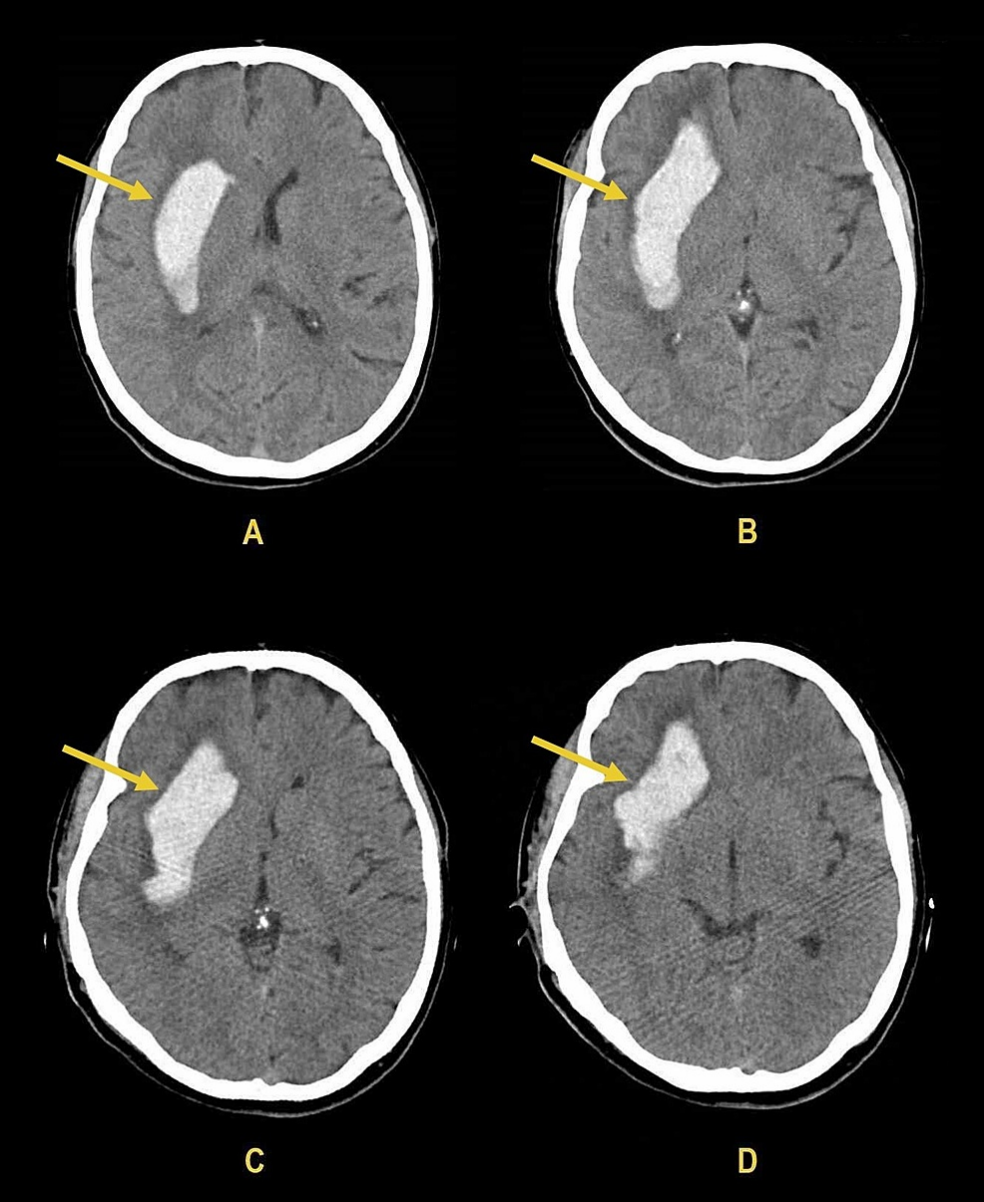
Intracerebral haemorrhage with associated midline shift of 4 mm to the left side on computed tomography scan of the head
